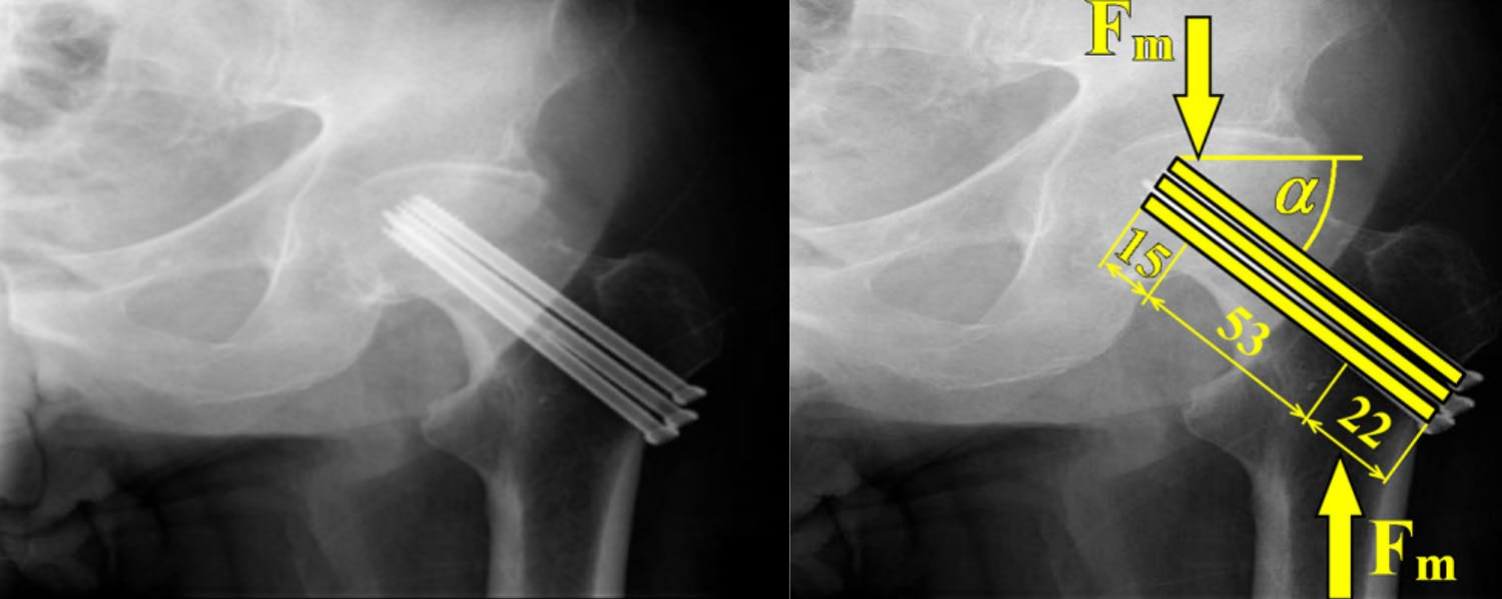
Three cancellous screws (X-ray image) and their approximation via parallel beams on an elastic foundation
